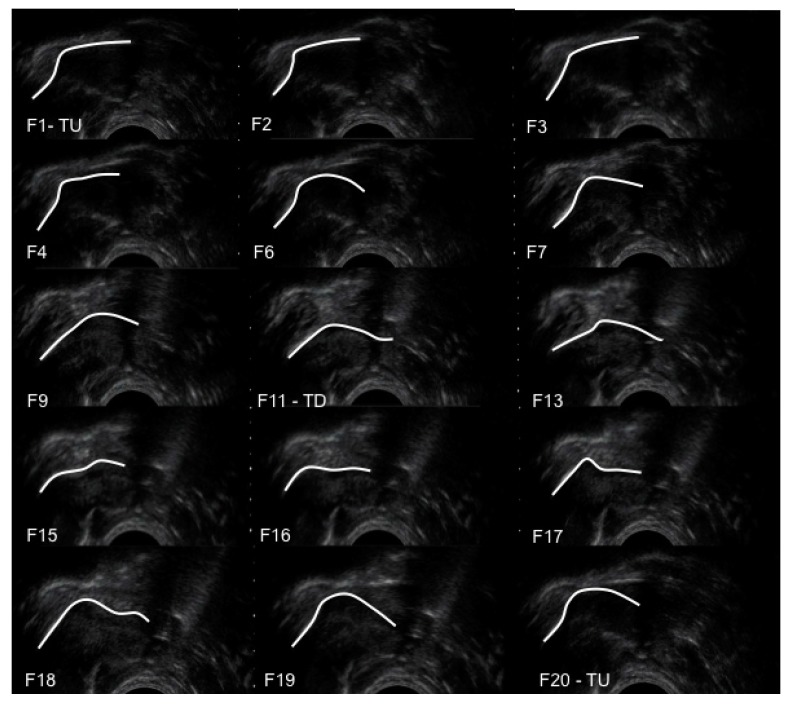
Sequential submental ultrasound frames (F1 to F20) of the infant intra-oral cavity with the tongue contour marked in white. TU, tongue up; TD, tongue down.
